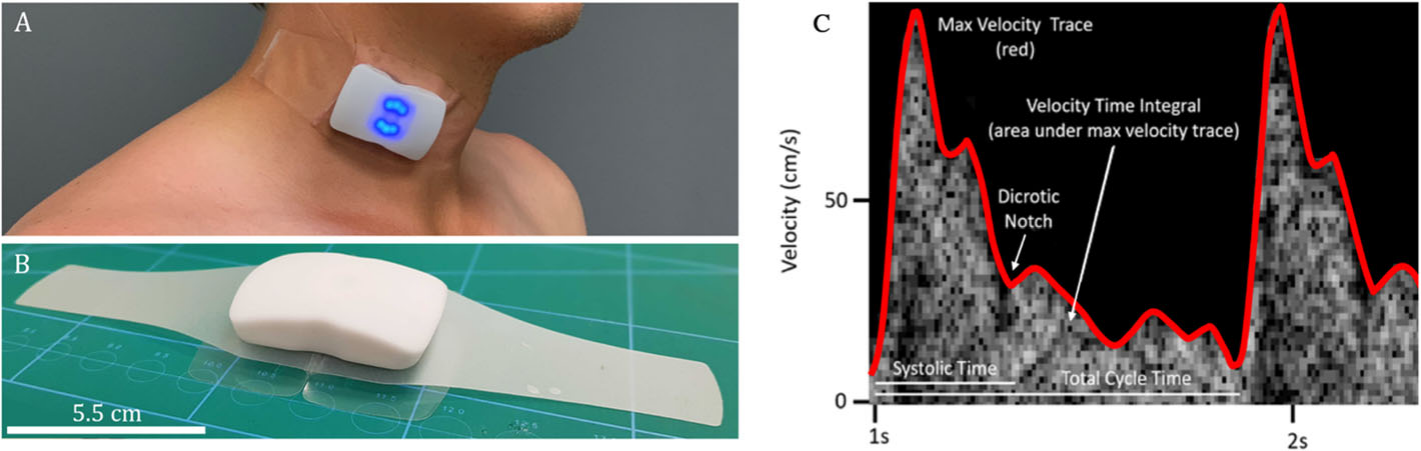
The wireless Doppler ultrasound patch. a Wireless, hands-free Doppler patch placed over common carotid artery of a healthy volunteer. b Scaled image of the Doppler patch. c Metrics obtained from wearable Doppler
